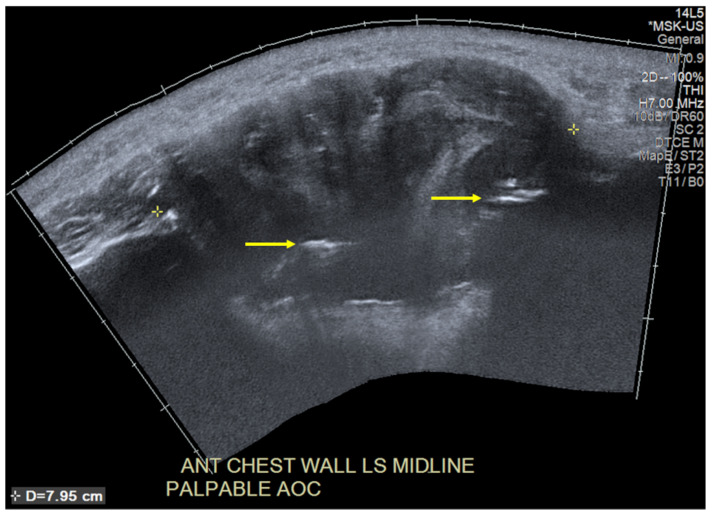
Targeted ultrasonography of the anterior chest wall shows a heterogeneous hypoechoic 7.95 cm mass encasing the sternal wires, which are seen as linear hyperechoic structures indicated by the yellow arrows. The sternum’s haematopoietic marrow and rich blood supply serve as ideal substrates for the development of tumours and infections. The majority of sternal lesions are malignant [1], with metastases being most common in adults.
